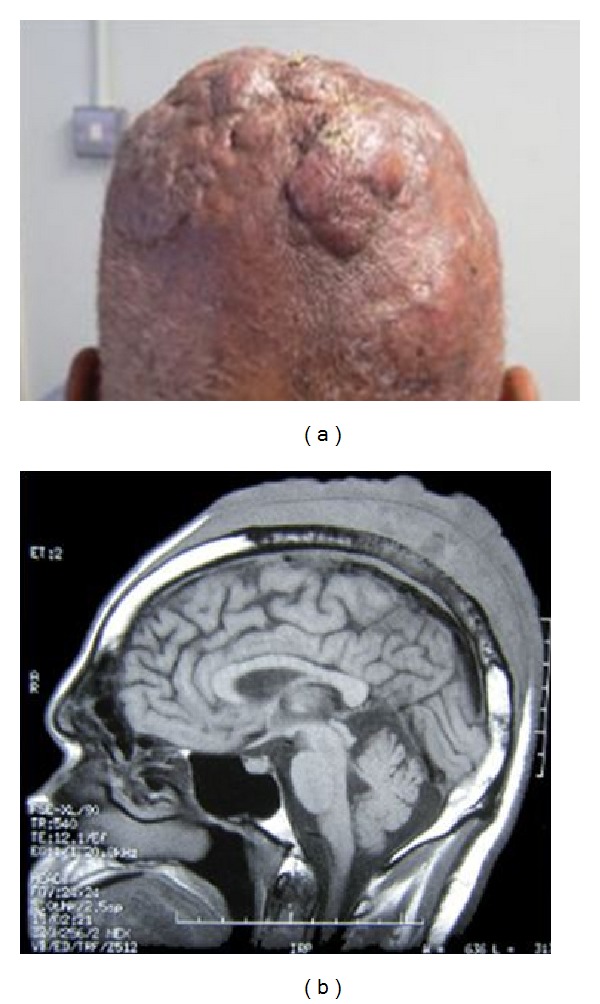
(a) Scalp nodules as seen at presentation. (b) MRI demonstrating an extensive mixed signal, soft tissue mass, over the vertex of the skull. There is infiltration through the inner and outer tables of the skull vault and extension into the dural membranes.
